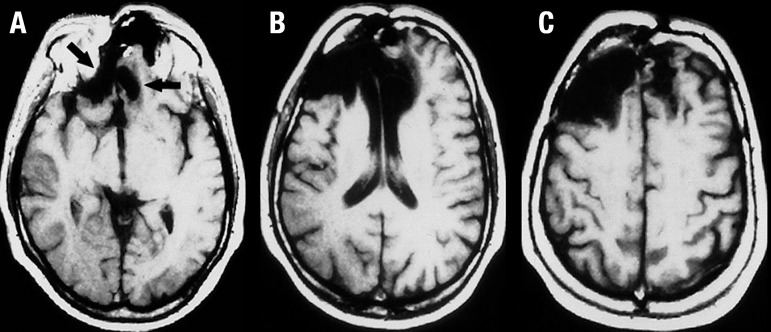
Axial brain MRI images. Axial T1-weighted images [A-C] demonstrate
